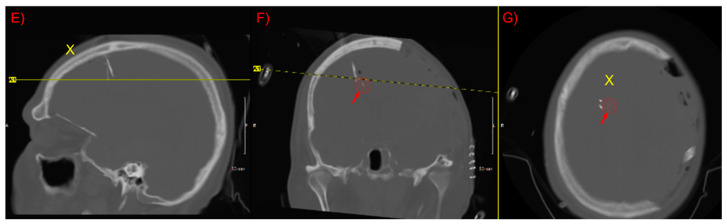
Computed Tomography (CT) head imaging. (A) Pre-operative imaging at presentation demonstrated left subdural hematoma with midline shift. (B–D) Postoperative imaging after decompressive hemicraniectomy and evacuation of the acute hemorrhage demonstrating peri-hematoma contusion but the resolution of the midline shift. An area of right temporal contusion is also seen with relative compression of the cisterns surrounding the brainstem. Intracranial monitoring devices were placed in the contralateral hemisphere approximately 2.5 cm under the inner table of the skull within the subcortical white matter. The location of the Bowman Perfusion probe is highlighted by a red circle. (E–G) Sagittal, coronal, and axial sections of the postoperative CT imaging windowed to highlight dense structures, including the intracranial monitoring devices. The Bowman Perfusion probe is highlighted with a red circle on coronal and axial sections. The approximate location of the DCS probe is marked with a yellow “X”.
